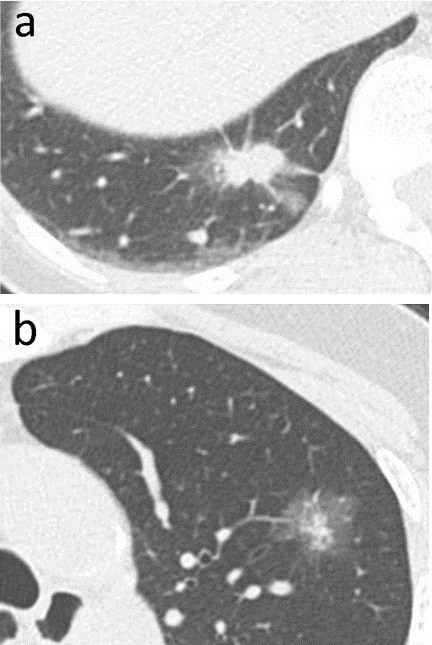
Preoperative chest CT image. It reveals a 38×12 mm stellate-shaped mass in the right lower lobe (a) and a 15×10 mm ground-glass opacity shadow in the left upper lobe of the lung (b).
